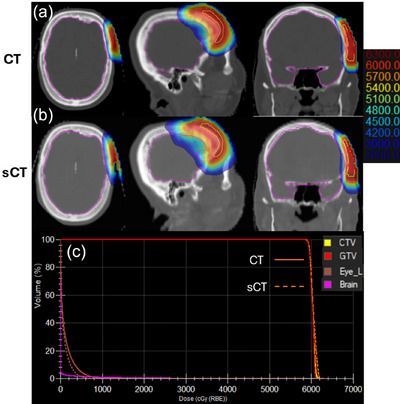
Dose comparison between planning CT and synthetic CT (sCT). (a, b) representative slices of dose distribution based on planning CT and synthetic CT for the basal cell carcinoma patient with MC algorithm, respectively. (c) The dose volume histogram comparison for targets and organs at risk.
